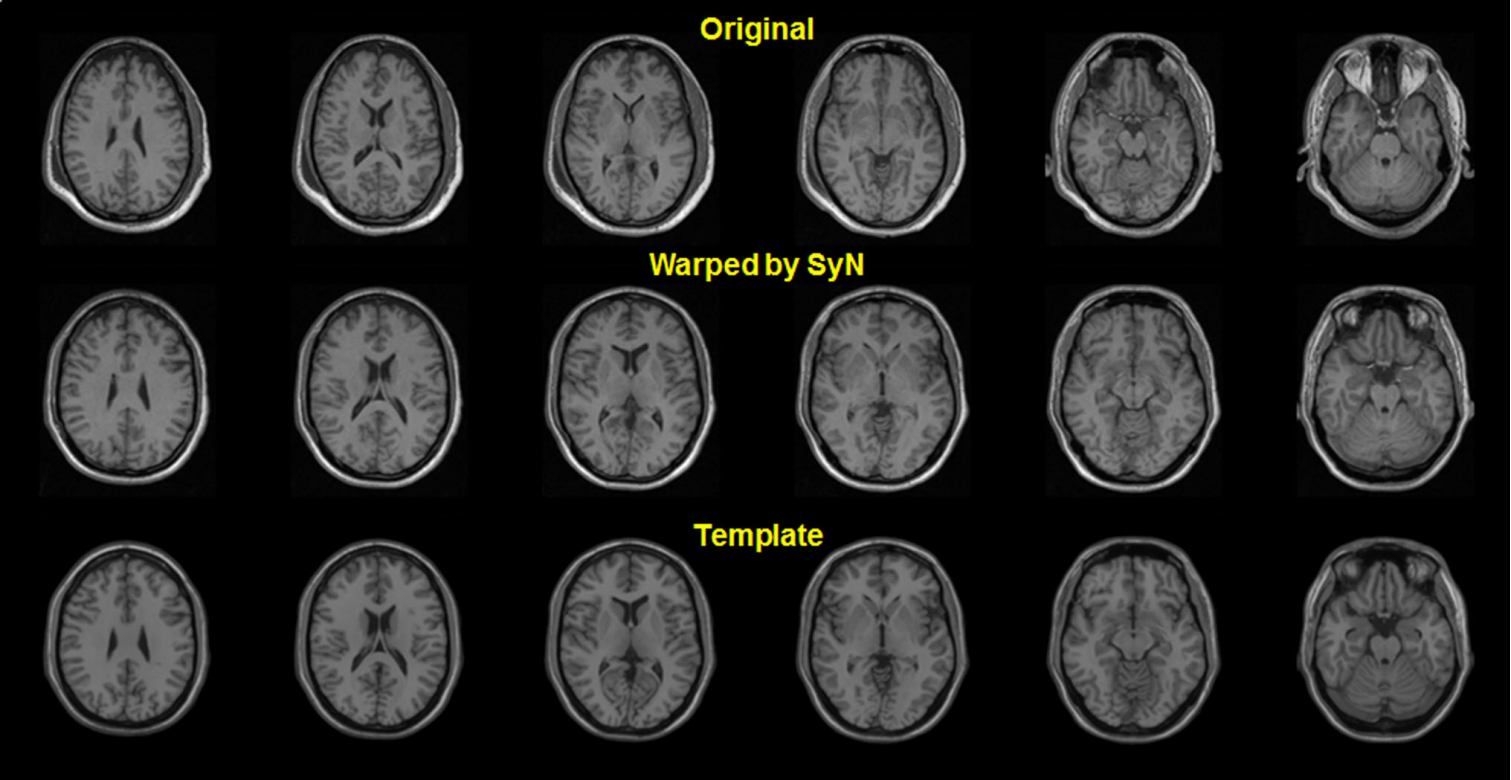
Comparison of the original image (*top row*), template (*bottom row*), and the warped original image to the template (*middle row*). Images are displayed in radiological convention. No focal lesion was observed in this subject (#2) based on the MR image
